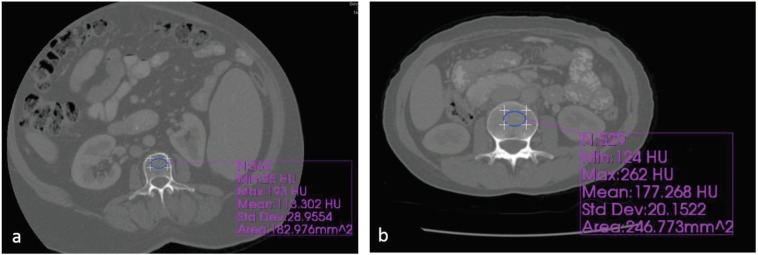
Contrast-enhanced abdominal CT scans showed density measurement from L3 vertebra on the axial plane. The
